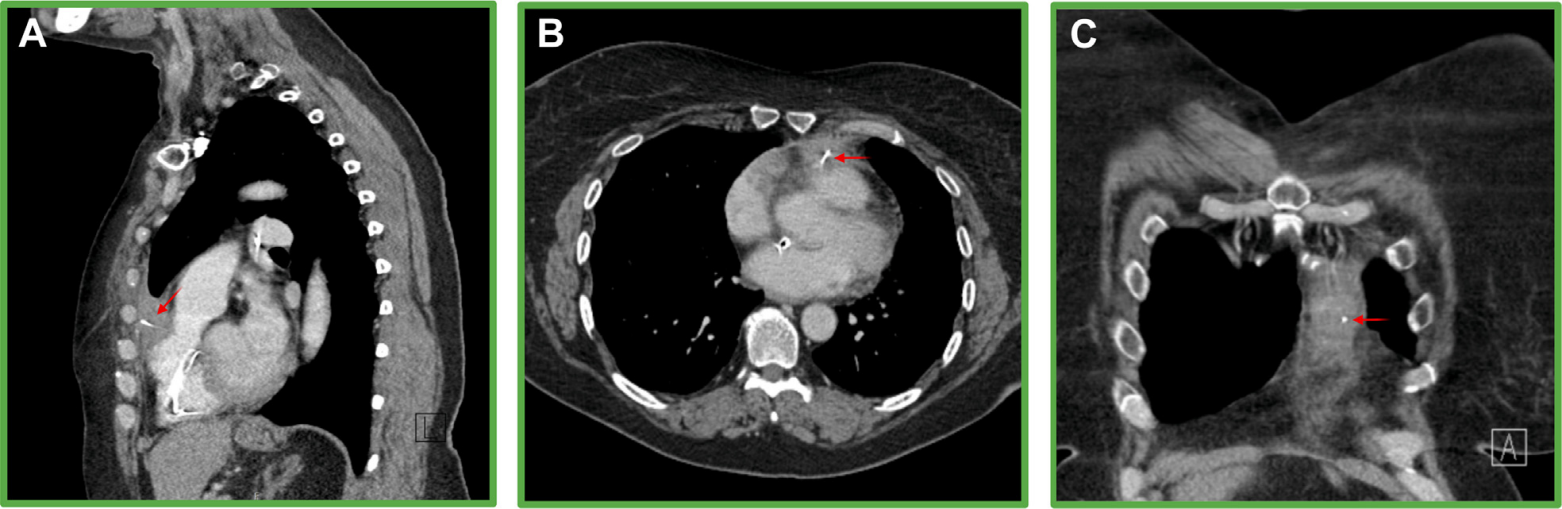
Anterior Course of the Wire, With 3 Separate Still Images Taken From Computed Tomography of Different Slices In each respective view, the red arrow details the course of the wire within the thorax. (A) Sagittal thorax slice. The wire can be seen coursing through the right ventricular outflow tract through the pericardium, through the subcutaneous layer of chest wall coursing superiorly to lie in the left anterior superior chest wall. (B) Transverse thorax slice. The exit point of the wire from the right ventricular outflow tract into the pericardium. (C) Coronal thorax slice. This view helps visualize how high the final position of the wire is in the left anterior chest wall.
